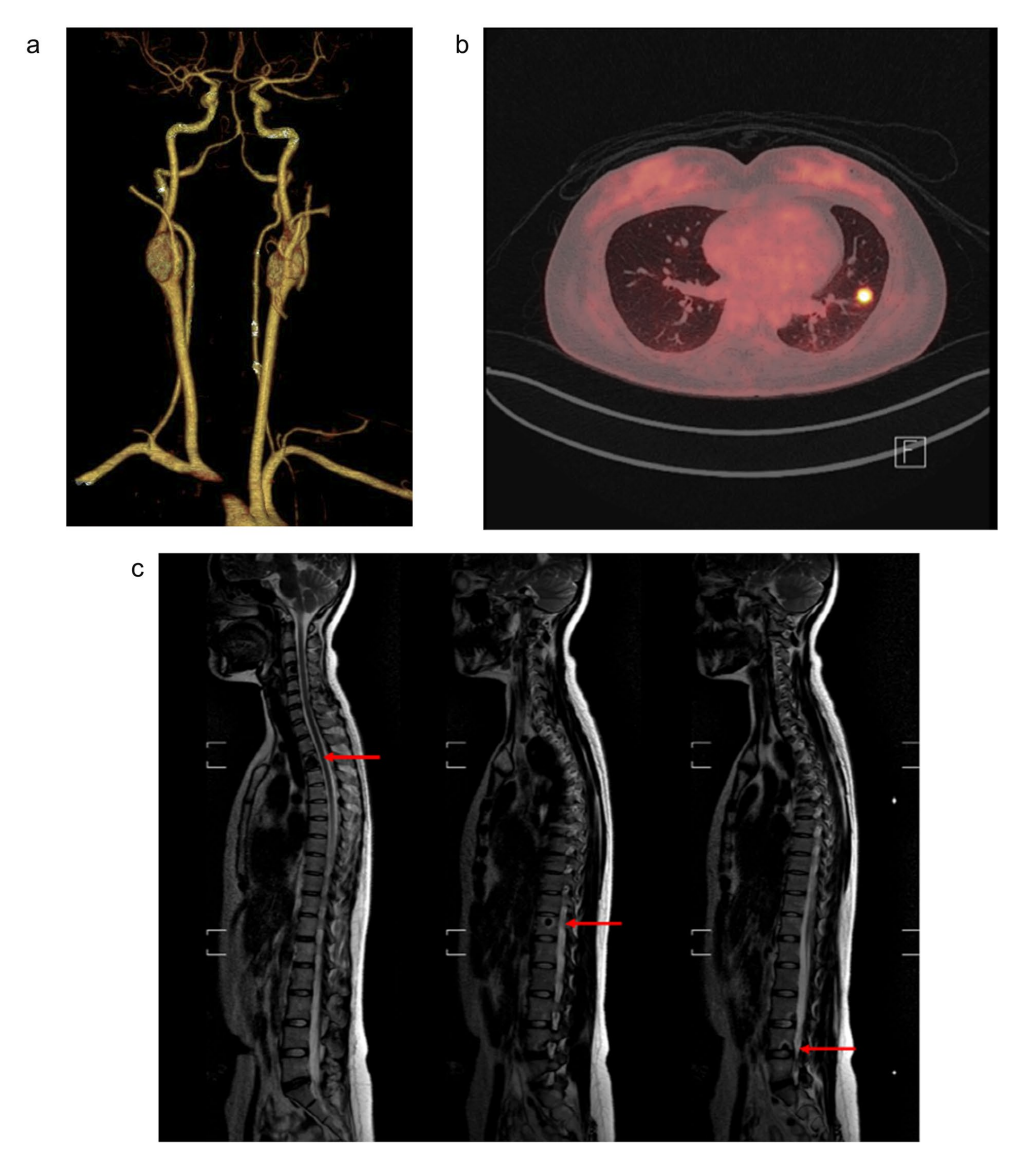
Bilateral carotid body paragangliomas (CBPs) with breast, spine, and lune metastasis (a) Head and neck computed tomography angiography reveals 26.4 × 16.5 mm and 25.2 × 17.3 mm sized right and left CBPs, respectively. (b) The large hyper-enhancing solid nodule at the left lower lobe of the left lung (12 mm) on positron emission tomography computed tomography presenting as metastasis of paraganglioma (c} Several different-sized sclerotic lesions in the thoracolumbar spine on spinal magnetic resonance imaging presenting as metastasis of paraganglioma
